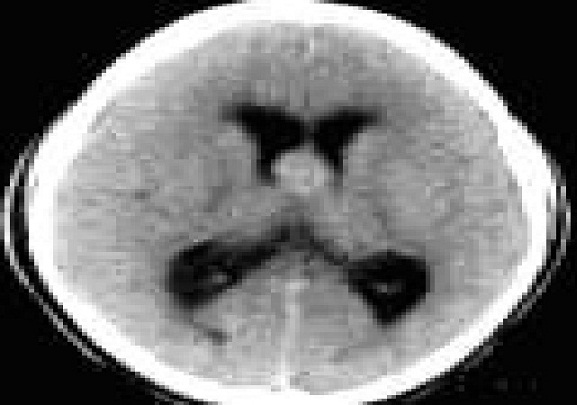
CT scan with an isodense nodular formation in the anterior part of third ventricle
